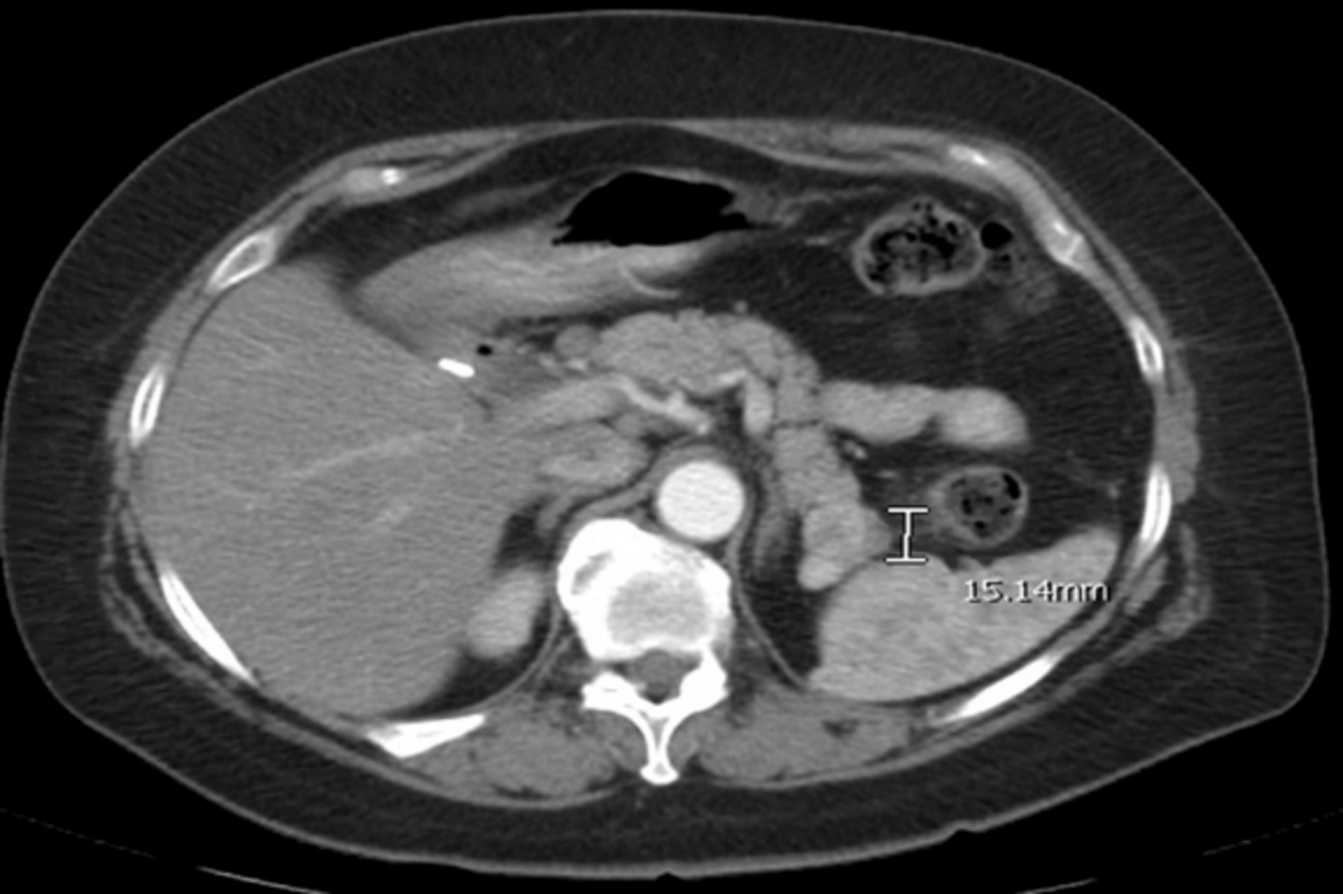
Year-eleven post-nephrectomy imaging showed interval increase of the pancreatic tail lesion. CT abdomen and pelvis demonstrated a mild increase in size of the mass lesion in the pancreatic tail. The lesion measured 0.9 cm previously, experienced an interval increase in size, and then measured about 1.5 cm.
